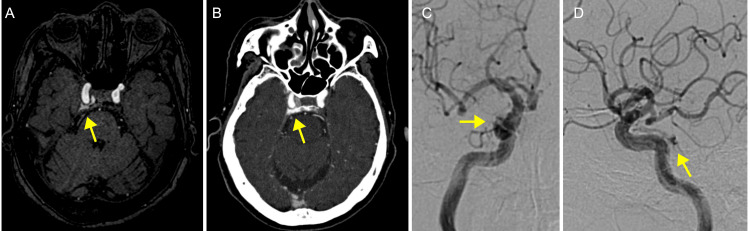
CS-dAVF (A) Magnetic resonance angiography demonstrated an abnormal signal confined to the right cavernous sinus (arrow). (B) Contrast-enhanced computed tomography demonstrated enhancement of the right cavernous sinus (arrow). (C-D) Cerebral angiography (selective right internal carotid angiography) revealed a shunt from the right meningohypophyseal trunk to the posterolateral portion of the right cavernous sinus (arrows).
